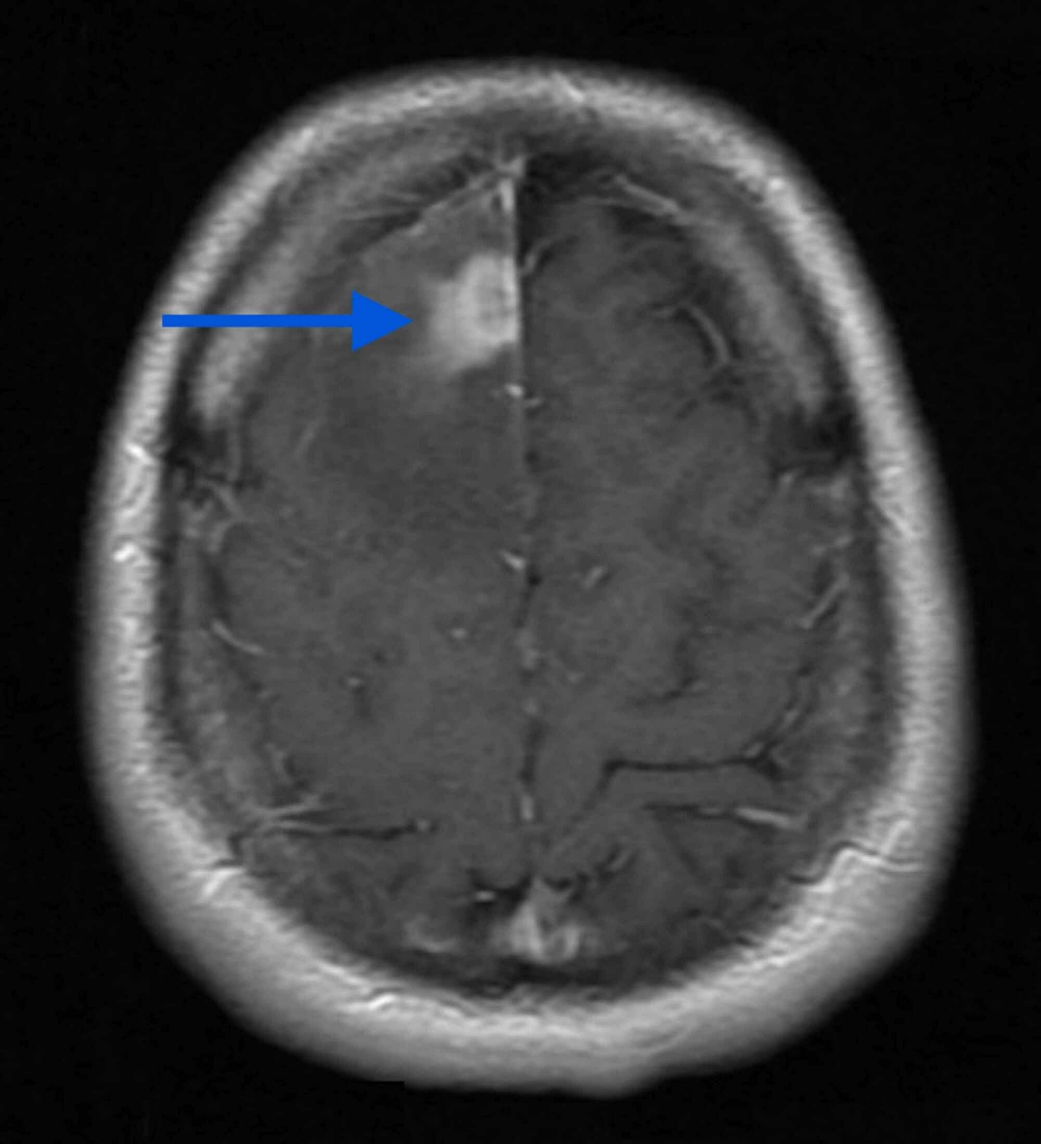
Axial post-contrast T1 MRI showing 16×14×21 mm conglomerate ring-enhancing lesion in the paramedian region of the right frontal lobe with surrounding edema.
